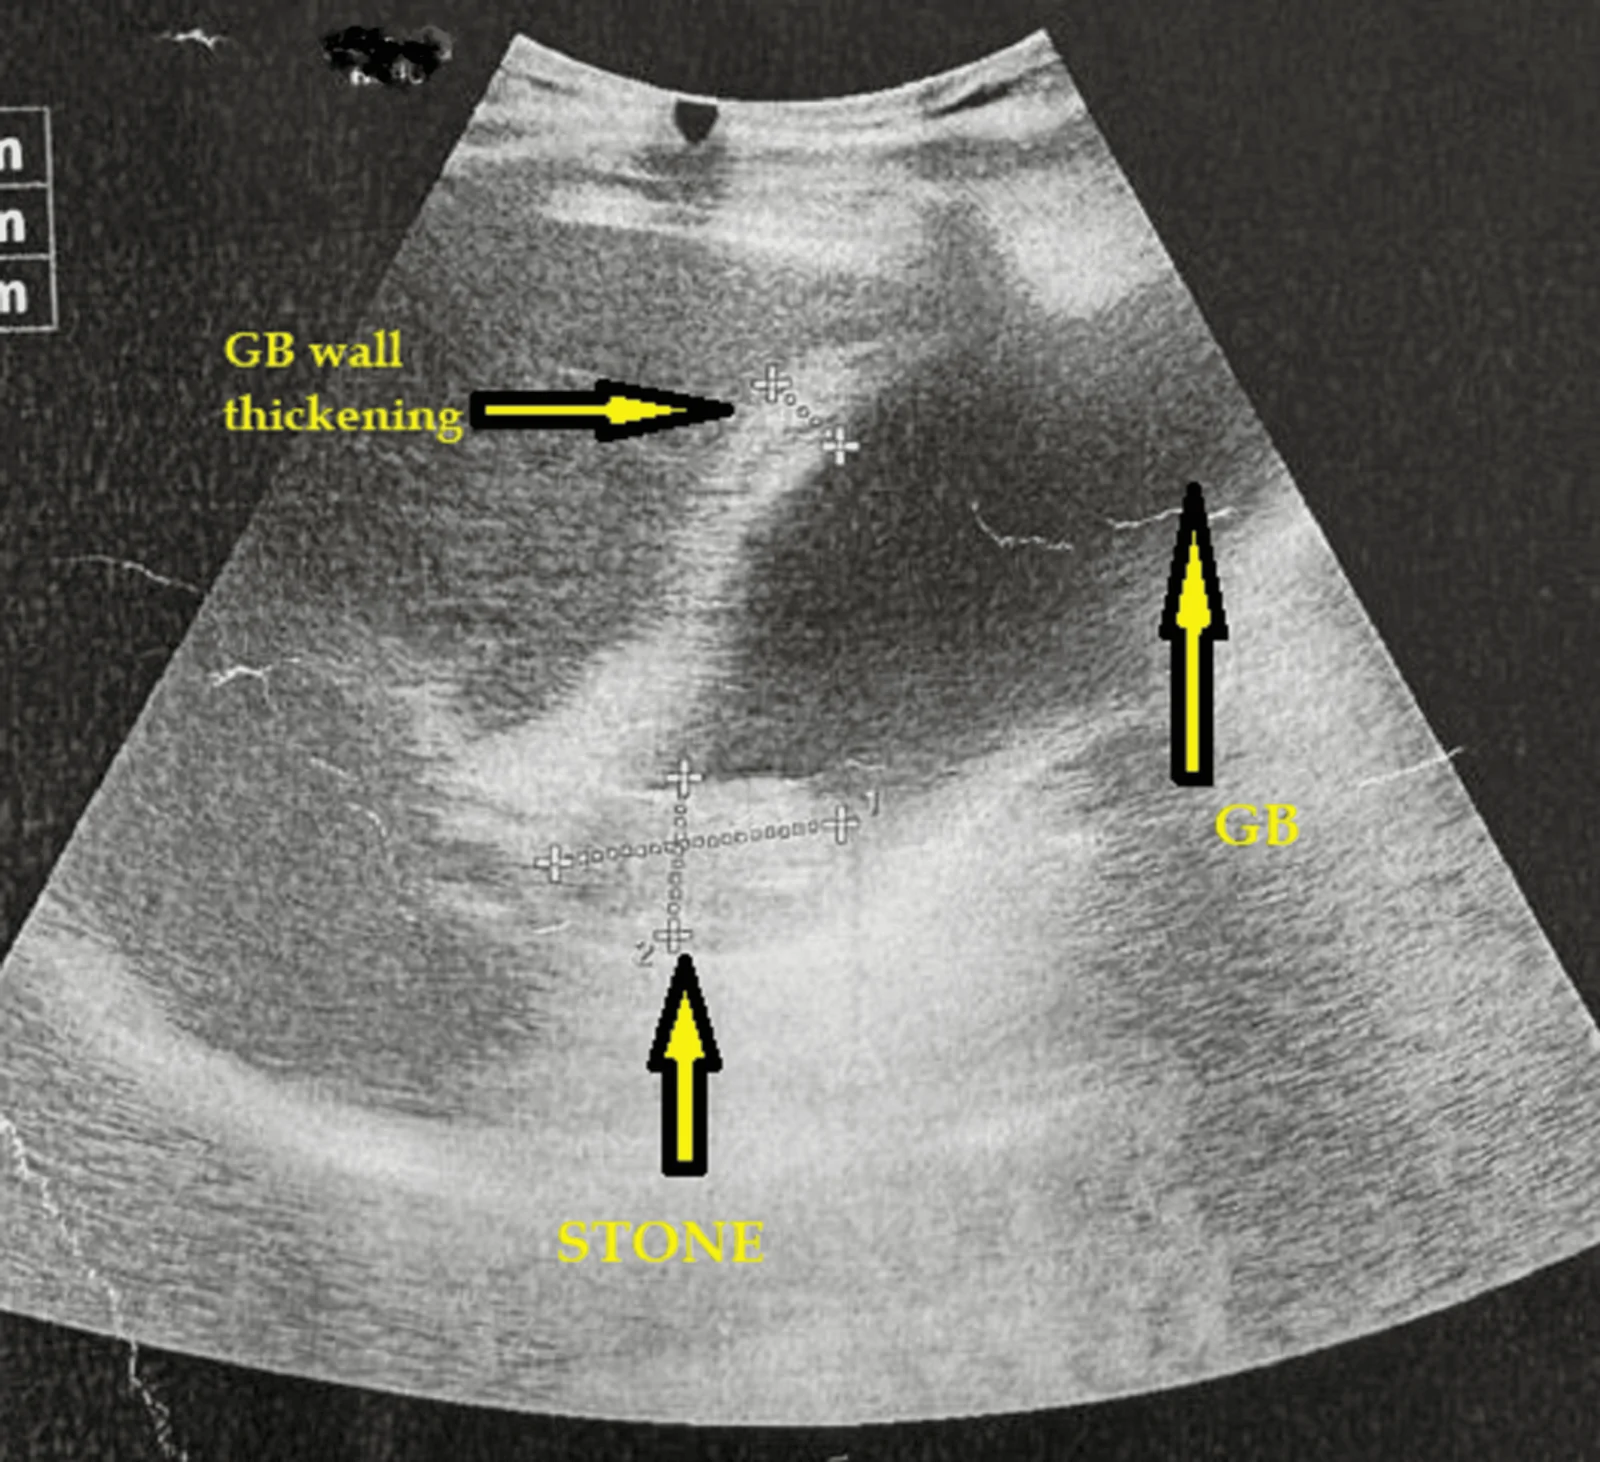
Ultrasonography showing gallbladder (GB) distension, wall thickening, and a stone at the GB neck.
